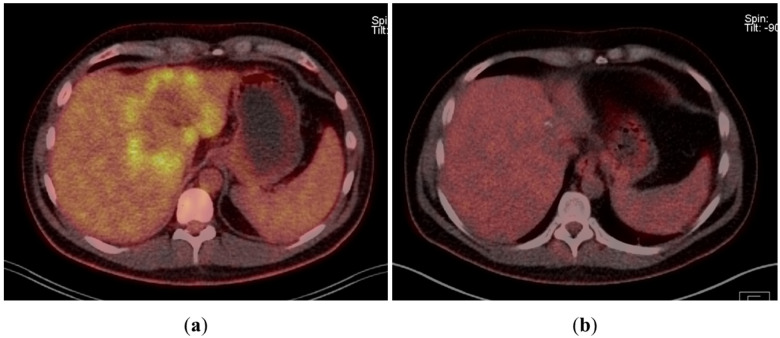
(a) Tumor in FDG-PET-CT scan at the time of initial presentation. (b) Tumor in FDG-PET-CT scan after chemotherapy and bilobar SIRT.
